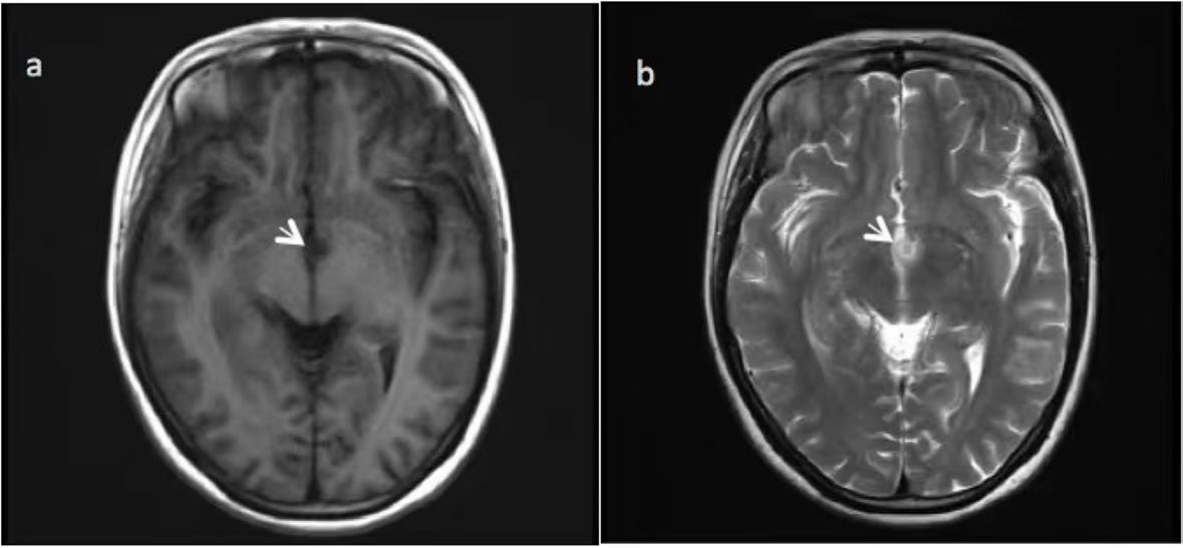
Postoperative cranial MRI T1WI (a) and T2WI (b)
